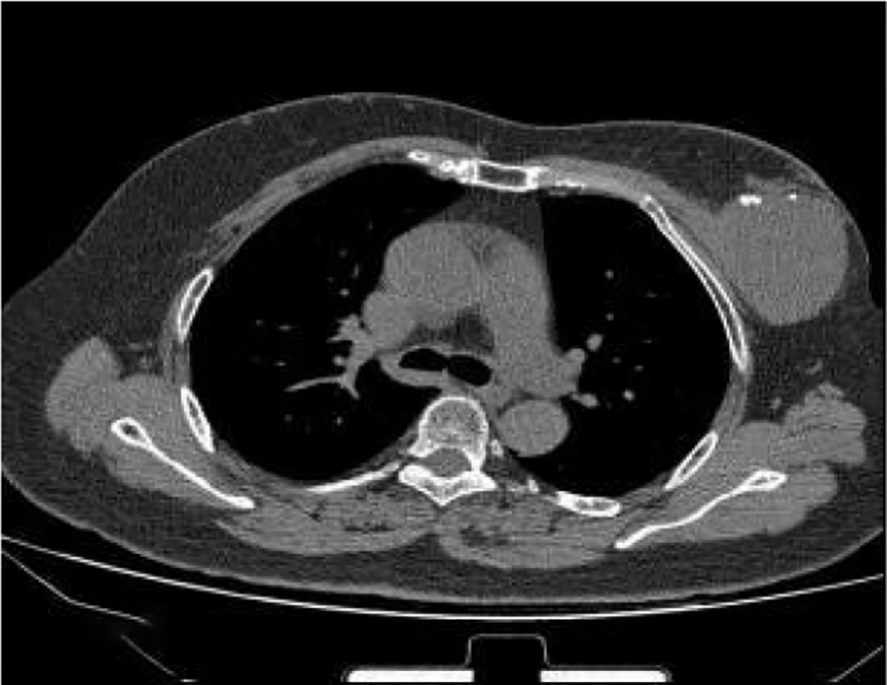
Magnetic resonance imaging revealed a round mass with smooth margins and internal punctate dense shadows.
